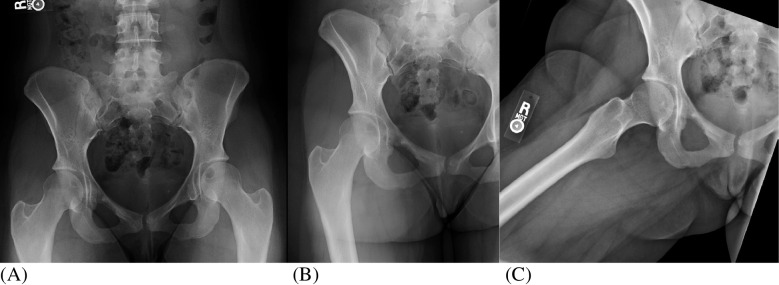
Hip and pelvis radiographs. A AP pelvis and B, C AP and abducted lateral views of the right hip radiographs demonstrate mild bilateral hip dysplasia without associated arthritis. There is a subtle lytic lesion in the right superior pubic ramus as well as an incidental incompletely assessed lucency within the left femoral head, which corresponds to the abnormality on subsequent MR images
